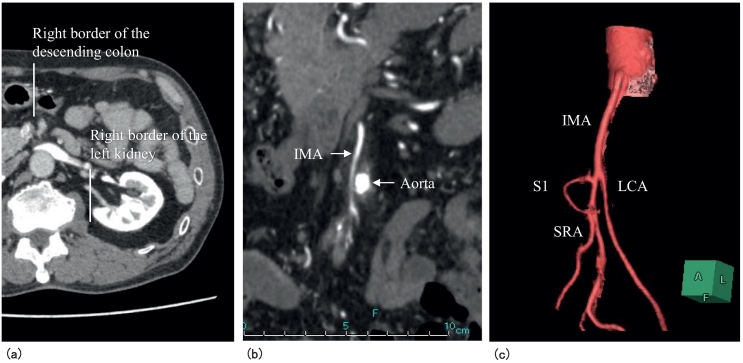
Diagnosis of PDM (contrast-enhanced thoracoabdominal CT). a) Horizontal section: The right margin of the descending colon was located on the right of the medial margin of the left kidney. b) Coronal section: The IMA course involved the right of the abdominal aorta. c) 3D angiography: The LCA, S1, and SRA radially branched at the same position from the IMA.
